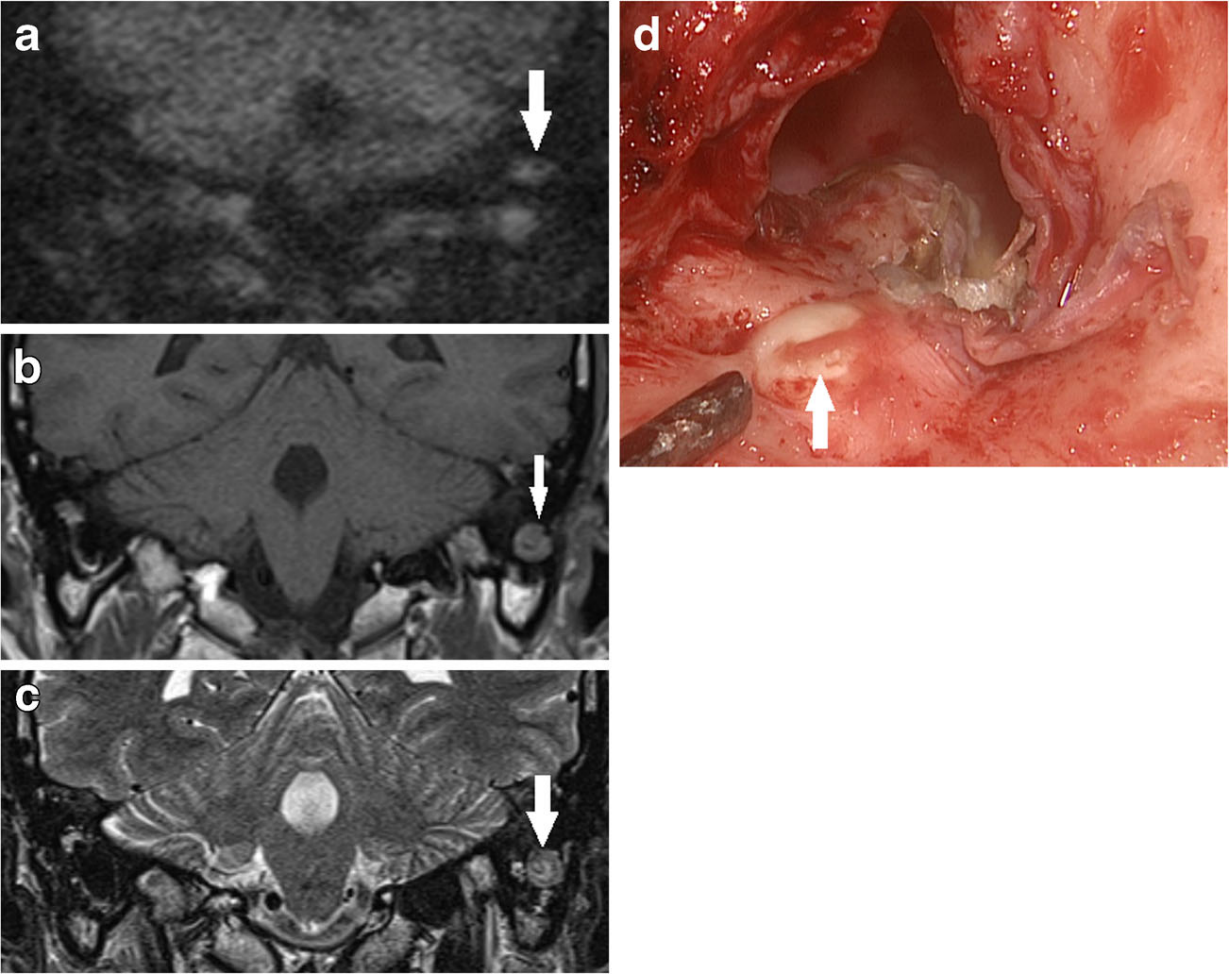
Using DWI following canal wall down surgery. a DWI b1000 image depicted residual disease (*white arrow*) at the mastoid tip, also seen as low signal on the b corresponding non-contrast enhanced T1 weighted image and a heterogenous, but predominantly high signal on the c T2 weighted image. d Surgical exploration confirmed residual cholesteatoma pearl (*white arrow*) walled off in the mastoid tip and was not readily visible on direct inspection at the preceding follow-up clinic. The lesion also had low ADC signal and value
